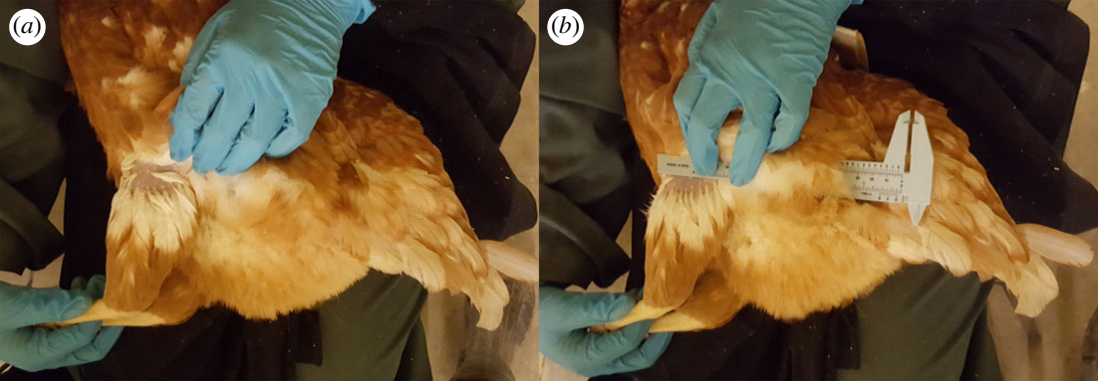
Placement of hens during ultrasound imaging of the lower leg. Hens were gently laid in the lap of a bird handler to image the left or right leg. The current figure shows placement for the left leg, the head of the hen is out of view at the top of the figure and the vent located in the right bottom of the figure. (*a*) The shank and lower leg were held such that they formed a 90° angle by holding the tarsometatarsus with one hand while the other hand gently parted the feathers. (*b*) A plastic vernier calliper was placed just below the knee and perpendicular to the length of the tibiotarsus and the transducer placed caudal to the calliper to obtain lower leg cross-section.
